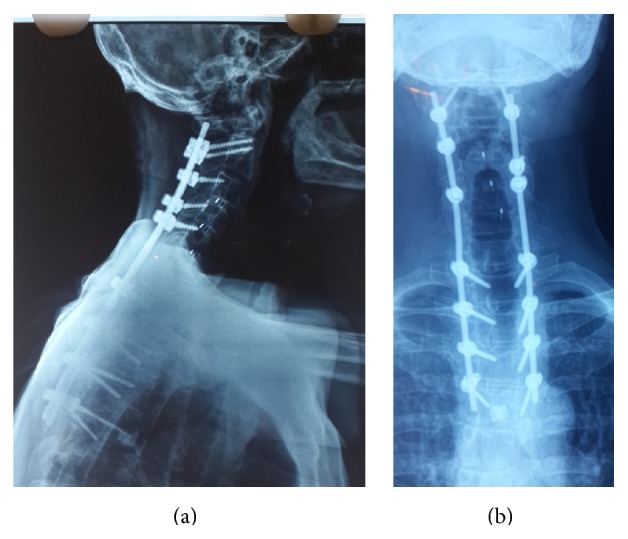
(a) Lateral cervicothoracic X-ray showing correction of the deformity 18 months after surgery. (b) Face cervical radiograph, indicating proper placement of the screws.
